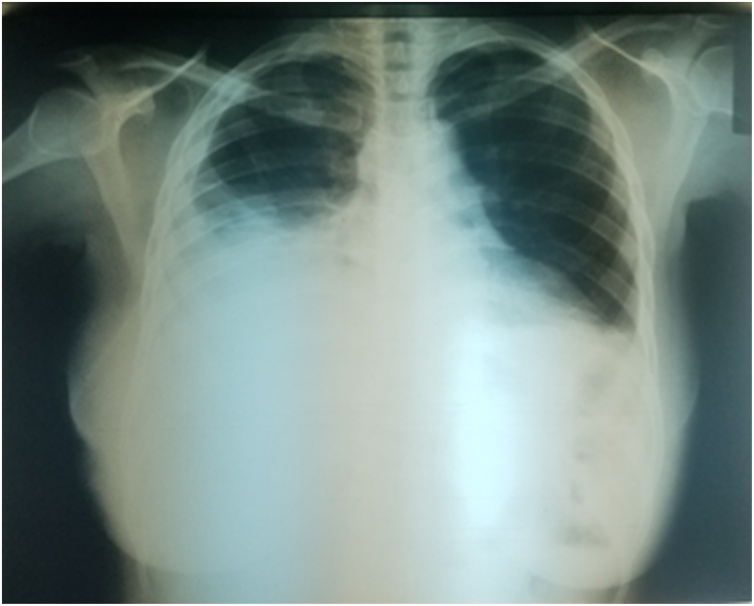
Chest X-ray at presentation showing massive right-sided pleural effusion.
